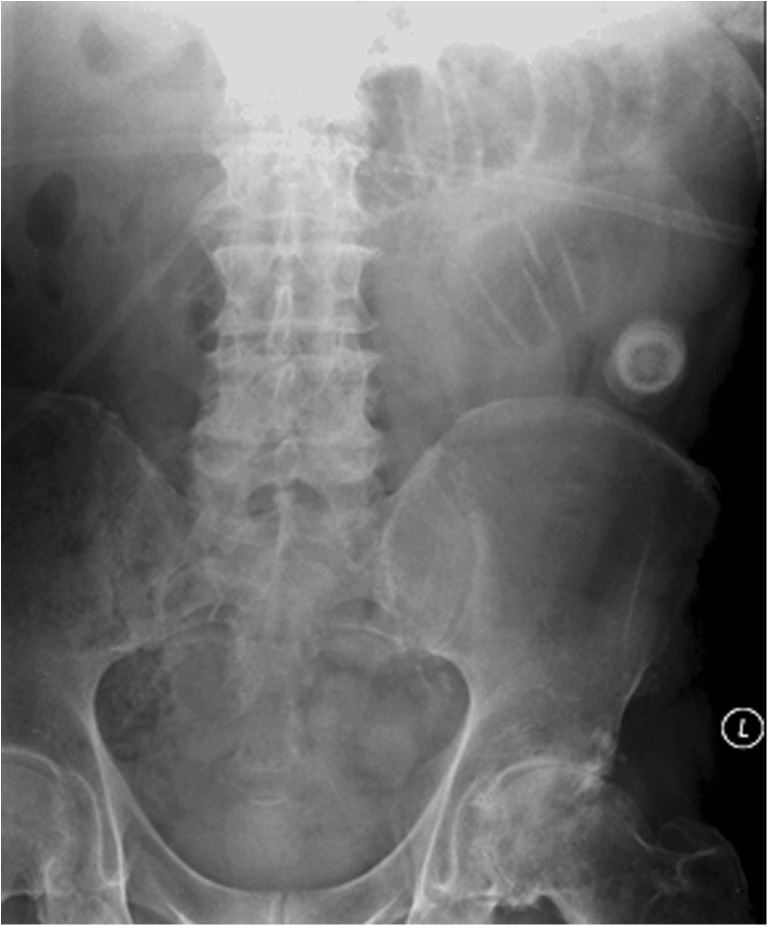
Plain abdominal film at time of admission. Poor quality plain abdominal radiograph obtained in the Emergency Unit, with only part of the abdomen examined. Note the foreign body in the left lower abdomen (ectopic gallstone), with left-sided loops of dilated small bowel. Nil convincing pneumobilia
